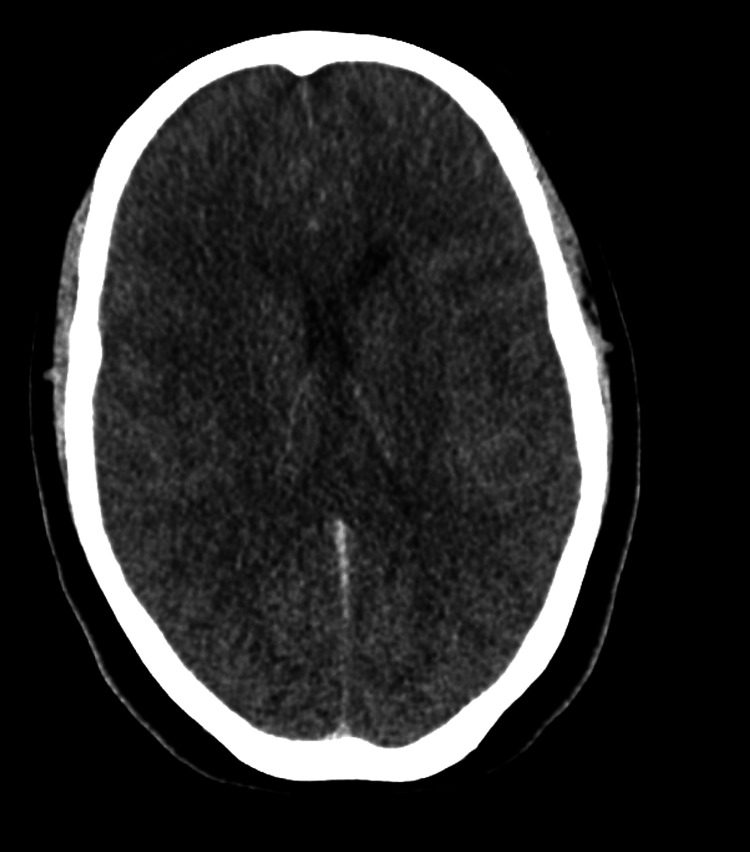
CT head superior slice Diffuse cerebral edema with sulcal effacement and cerebral/cerebellar swelling is observed, resulting in effacement of the lateral (posterior and temporal horns) and fourth ventricles, along with downward displacement of the cerebellar tonsils. There is increased density within the basilar cisterns and along the tentorium.
